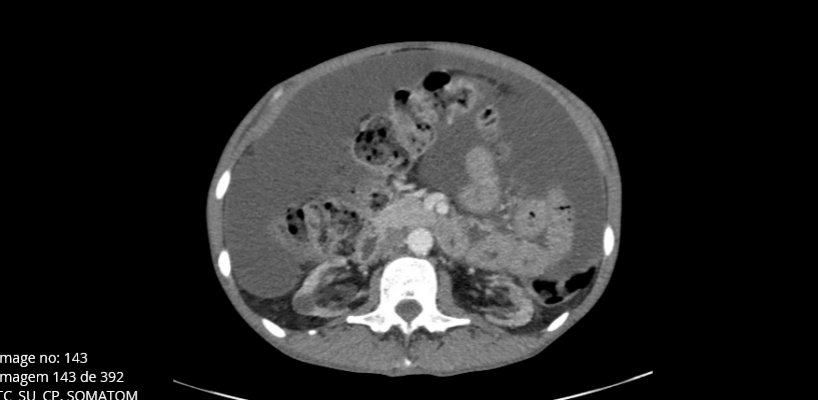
Ascites in abdominal CT raised the suspicion for encapsulating peritoneal sclerosis
